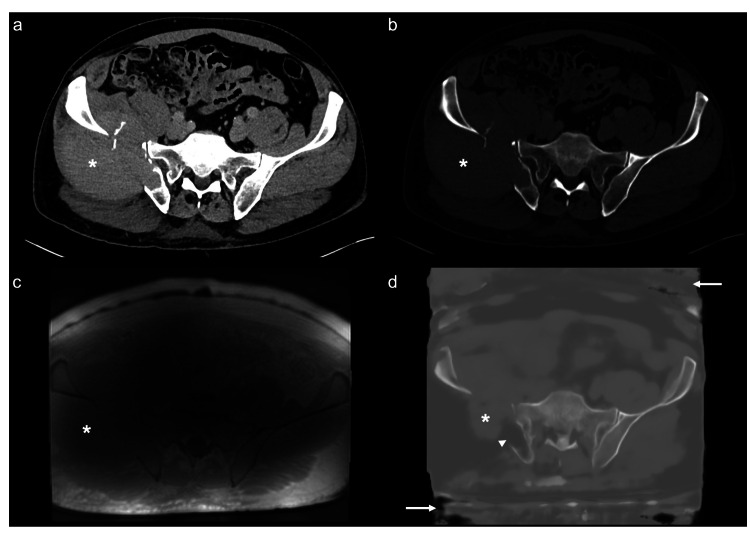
Exemplary CT (a, b), ZTE MRI (c), and pCT (d) images of the pelvis of a 54-year-old study participant. The pCT (d) image was generated from ZTE MRI (c) by applying a deep-learning algorithm. The images display a large tumor in the right iliac wing (asterisk; biopsy-proven plasmacytoma) which was underestimated in d since the model was trained with enhanced focus on bone regions. Nevertheless, lytic bone destruction by the tumor was correctly identified (arrowhead). Note the imperfect body masking (arrows) in d
